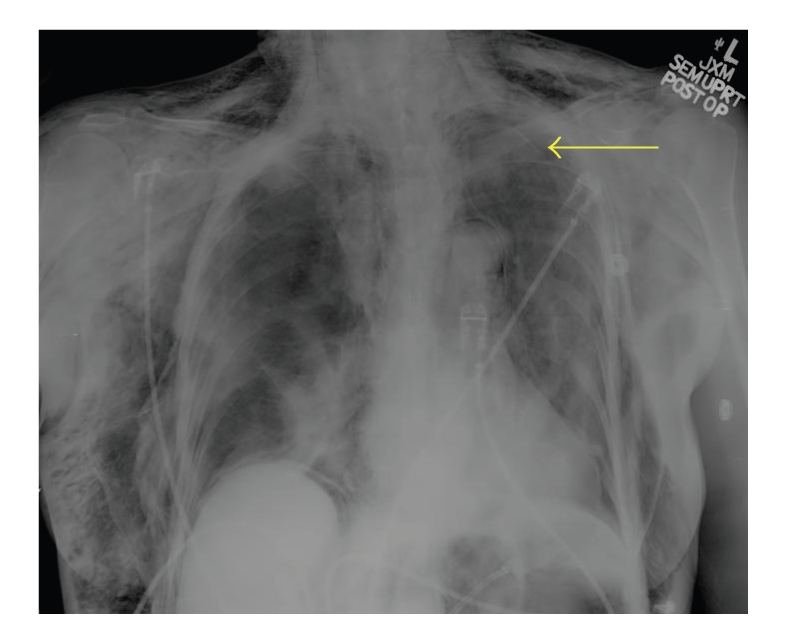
Portable chest X-ray showing subcutaneous emphysema, pneumomediastinum, and a left pneumothorax (arrow).
